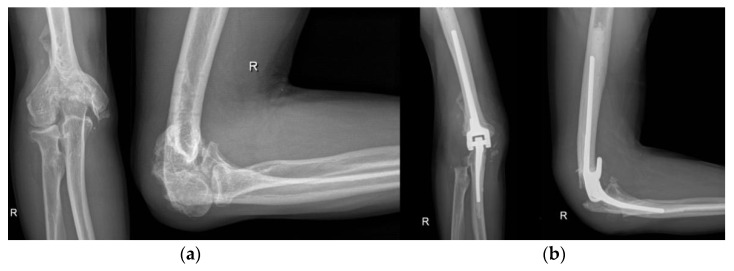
Total elbow arthroplasty. (a) preoperative X-ray; (b) postoperative X-ray after total elbow arthroplasty.
